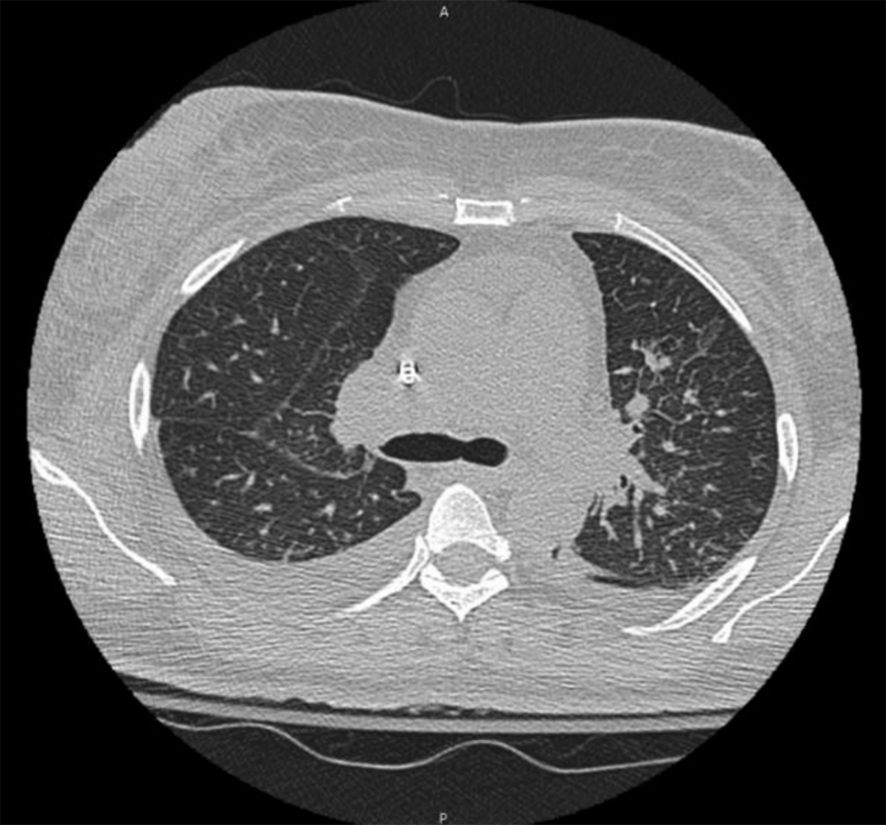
CT chest demonstrating a small right pleural effusion and moderate ground-glass opacities.
